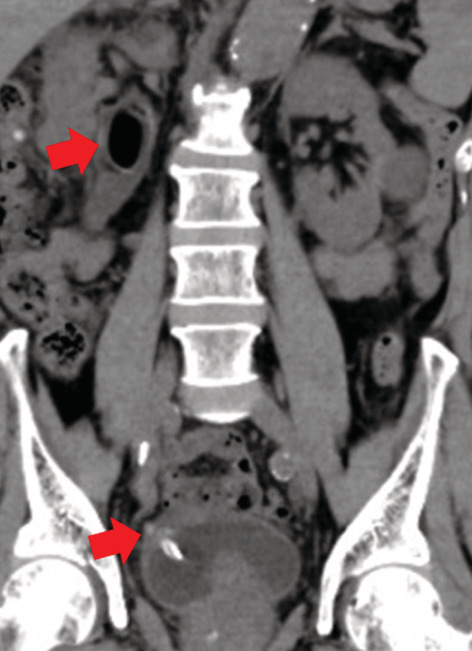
Non‐contrast‐enhanced computed tomography of the abdomen (coronal section) showing a bladder catheter embedded in the bladder wall (arrow) and showing air in the ureter (arrow)
